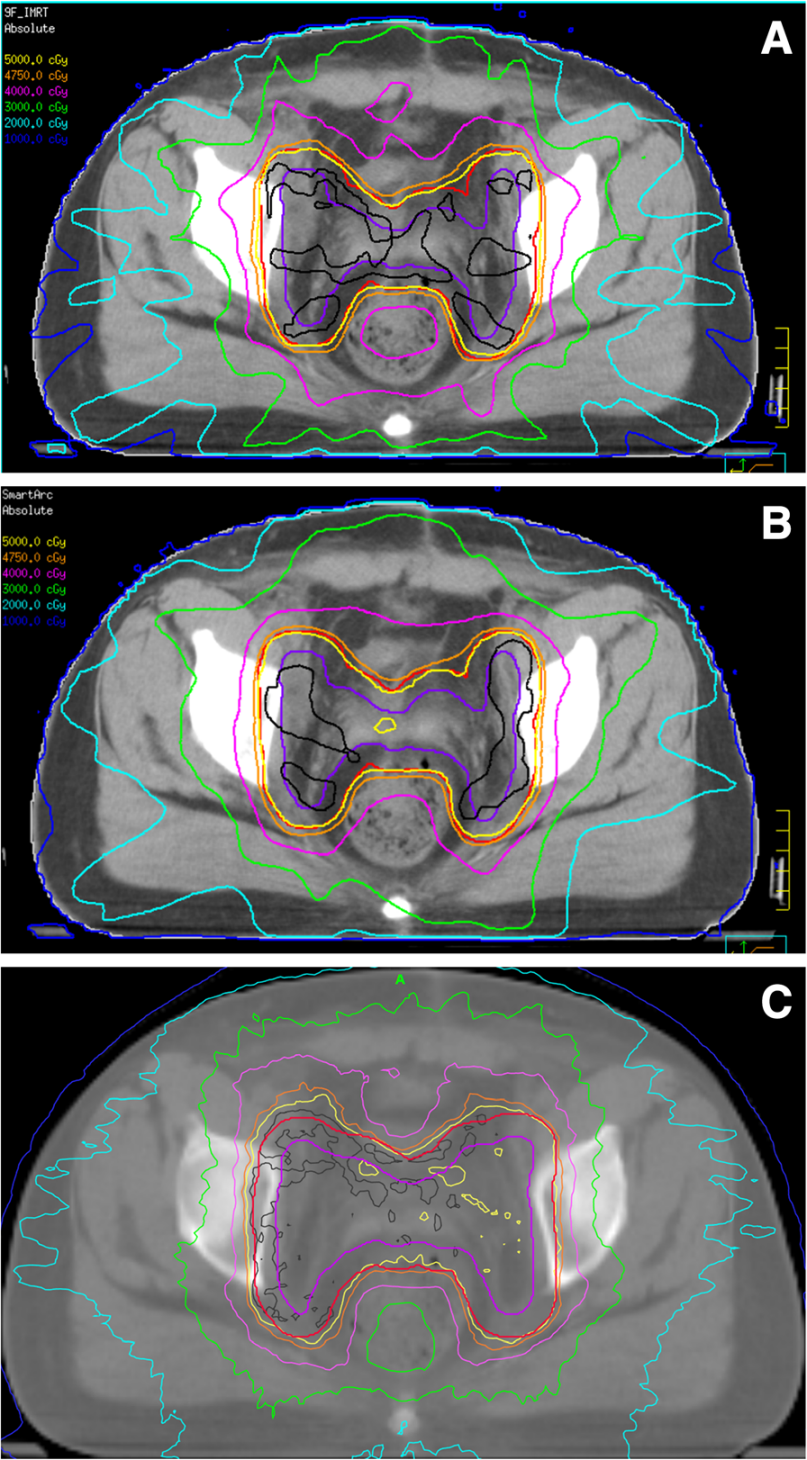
Representative axial computed tomography slices showing isodose distributions. (A) IMRT. (B) VMAT-S. (C) Tomotherapy. PTV is shown in red, CTV in slate blue. Isodose lines are indicated as follows: inverse grey, 5250 cGy; yellow, 5000 cGy; orange, 4750 cGy; purple, 4000 cGy; green, 3000 cGy; sky blue, 2000 cGy; and blue 1000 cGy.
